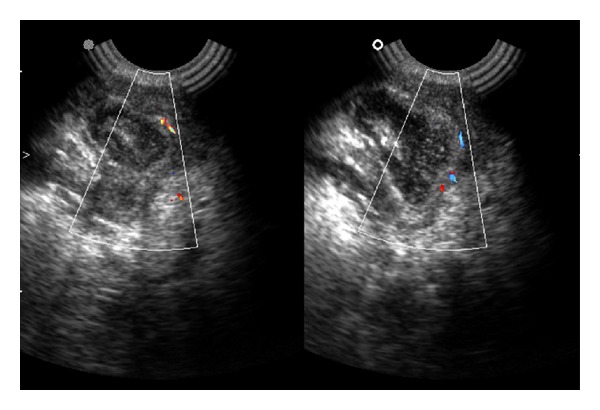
Color Doppler findings shows increased vascularity.
